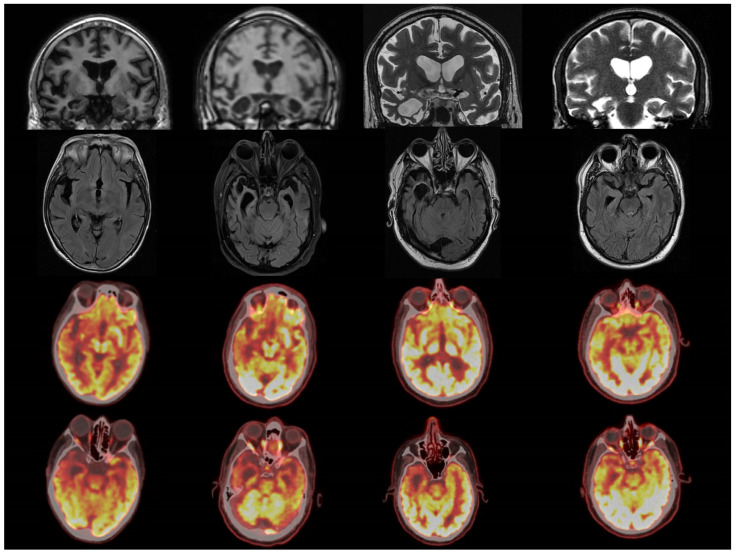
Concordance of structural changes on clinical MRIs and metabolic patterns on [^18^F] FDG PET-CT.
